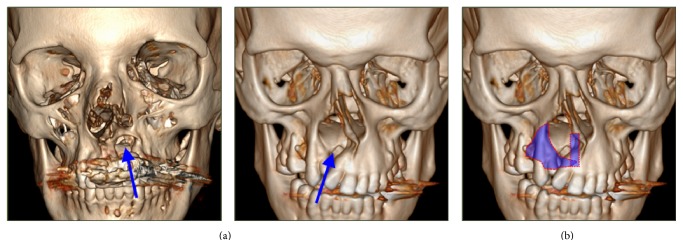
(a) Computed tomography pictures of two patients; arrows point to maxillary and piriform deficiency. (b) Computed tomography picture used to fashion the design of the cartilaginous construct (superimposed blue shapes).
